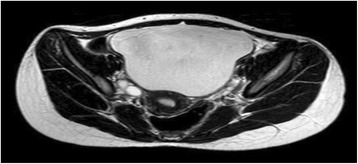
MRI imaging of a patient with spontaneous menarche
